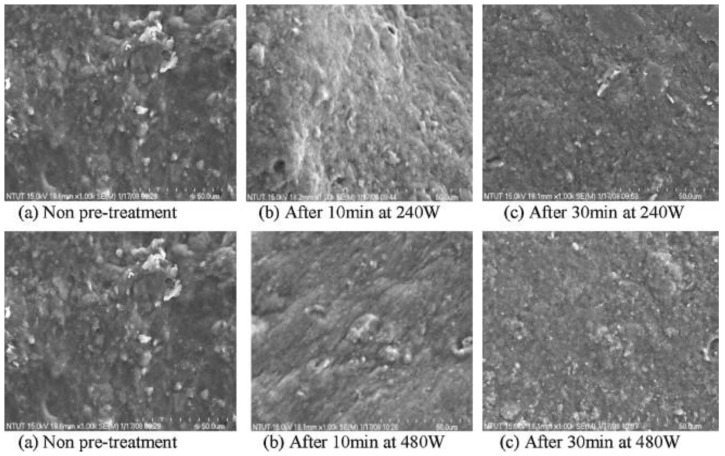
The morphology of the floc structure of sludge before and after ultrasonic pre-treatment under SEM [36]. Note: different lowercases represents food waste without ultrasound treatment (a), with sonication for 10 min (b) and with sonication for 30 min (c).
